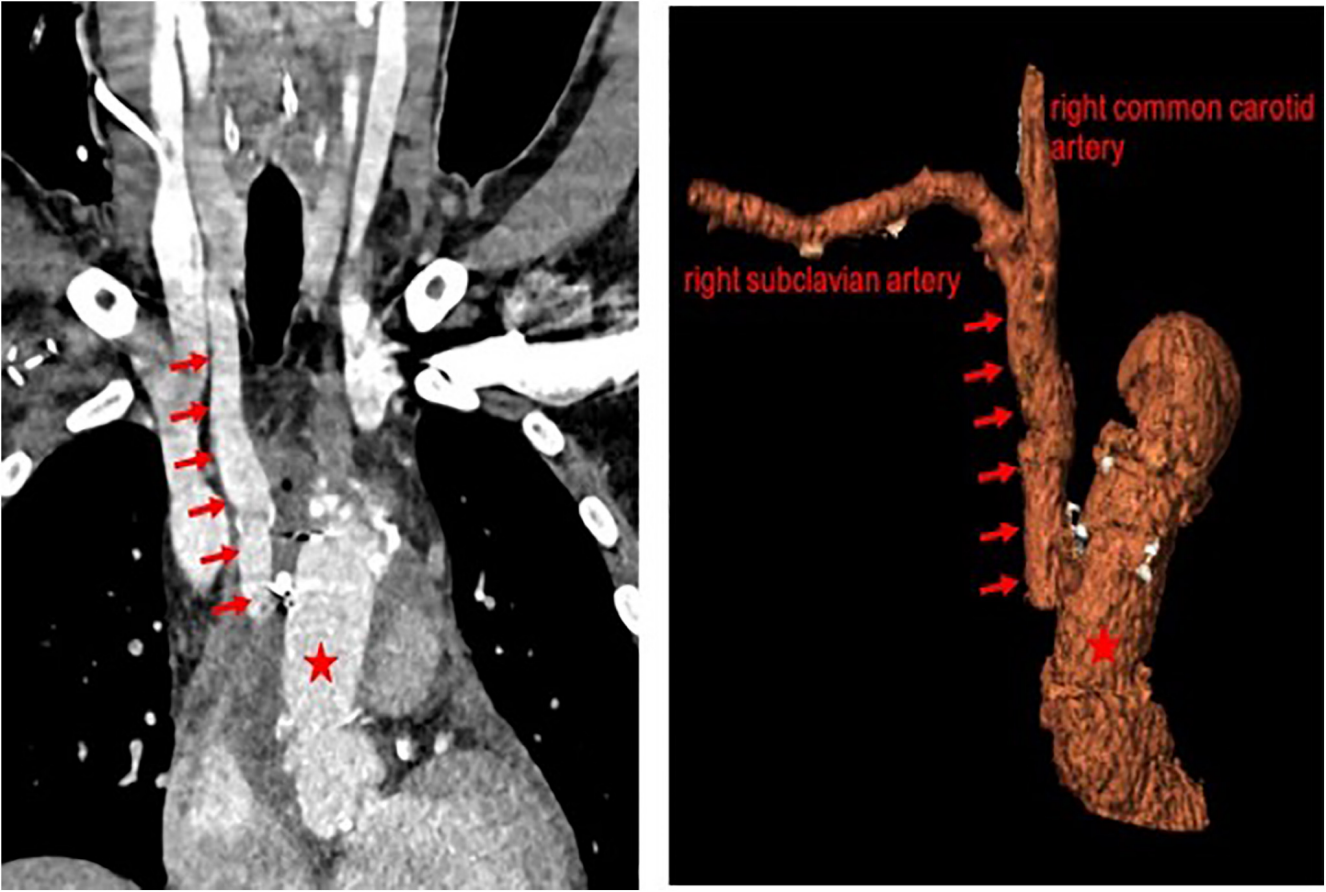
Postoperative computed tomography imaging and 3-dimensional reconstruction showing revascularization of the proximally occluded innominate artery with a vascular graft (*arrows*) between the ascending aorta (*star*) and the bifurcation of the innominate artery.
